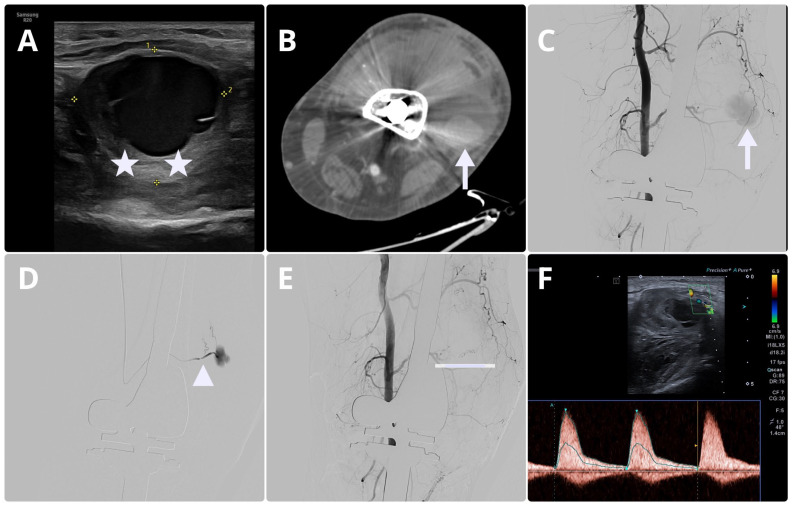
Baseline B-mode ultrasound demonstrated a partially thrombosed pseudoaneurysm (thrombosis visible in the lower part of the lesion—white asterisks) originating from the lateral branch of the popliteal artery (A). Doppler modality showed slow filling of the lesion. The diagnosis was further confirmed by computed tomography angiography (CTA), which disclosed the presence of a partially filled lesion at the distal part of the branch of the genicular artery (white arrow) (B). Initial angiography via a catheter placed in the popliteal artery revealed a pseudoaneurysm arising from the superior genicular artery with delayed filling of contrast (C) (white arrow). A microcatheter was then positioned, and embolization was performed (D) (white triangle—tip of the microcatheter). Control Digital subtraction angiography (DSA) confirmed complete occlusion of the lesion with no residual filling (white line—coils) (E). Follow-up ultrasound showed further thrombosis and reduction in size, with preserved flow in the proximal segment of the artery (F).
